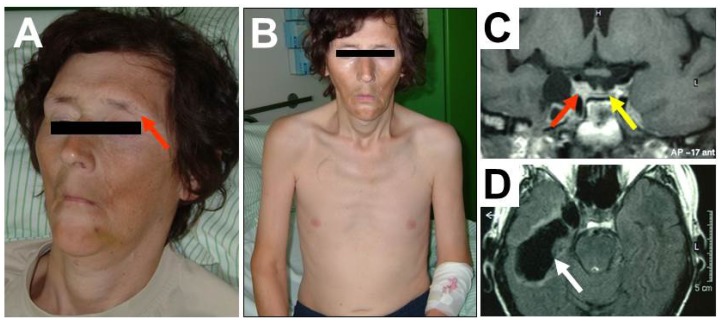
Forty-nine year-old patient with severe posttraumatic hypopituitarism. Clinical appearance, showing lack of facial hair, dehydration, Queen Anne’s sign (panel A), pale skin, muscular atrophy, loss of body hair and anorexia (panel B). T1-weighted coronal MRI of the pituitary region, with flattened pituitary gland at the bottom of the sella (panel C, yellow arrow) and contusional defects in the temporal lobe (panel D, white arrow). Tissue adjacent to the right cavernous sinus (panel C, red arrow) compatible with dislocated pituitary tissue after fracture of the middle cranial fossa.
